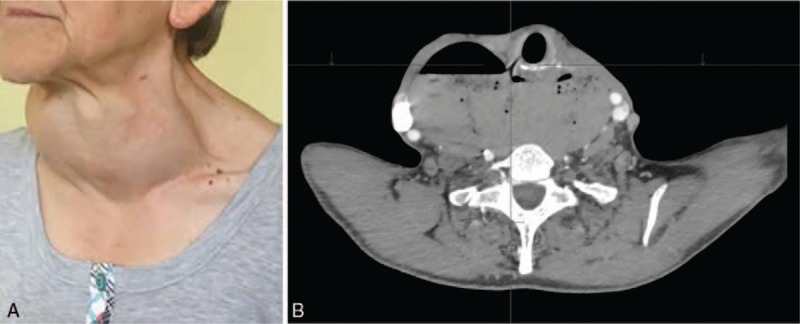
(A, B) Sixty three-years old women with the diverticulum with 15 cm long septum and esophgo-tracheal fistula. The diverticulum was permanently visible even she vomited and emptied it, and mimicked a large goiter. Computed tomography (CT) image of this large diverticulum with visible fistula between the esophagus and the trachea, which is abnormally translocated by the diverticulum permanently filled with air.
